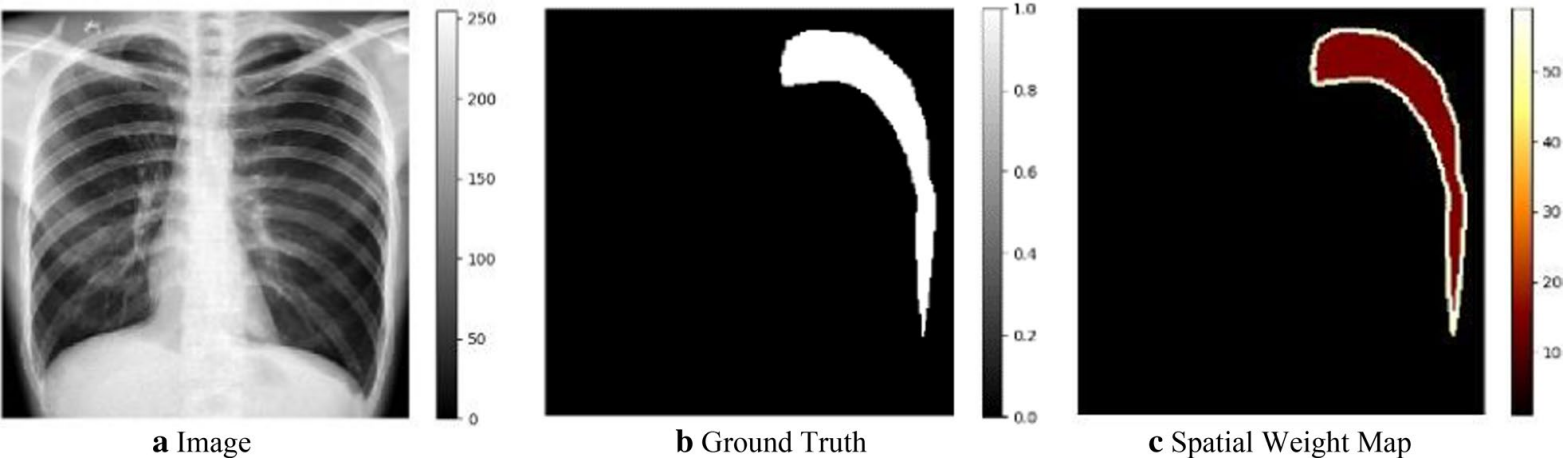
The process of generating the spatial weight map. The groud-truth image b is delineated by radiologist according to the chest X-ray image a. Through edge detection and morphological dilation of the boundary contour pixels of the target class, the spatial weight map c can be generated from the ground-truth image b. The colors in the spatial weight map represent the weight distribution according to its relative class frequency
